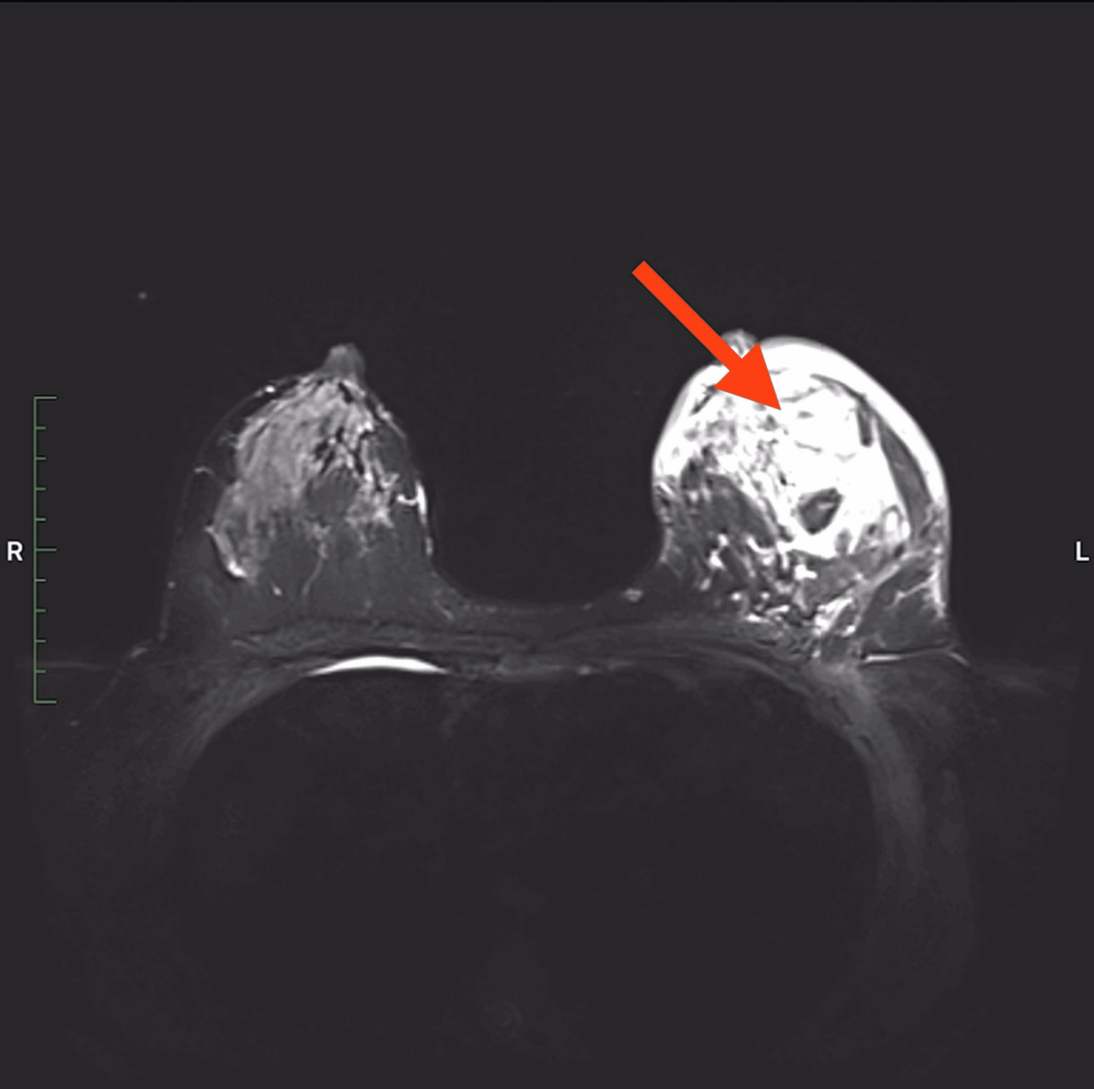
MRI of both breasts showing abscess in the left breast (red arrow) MRI: magnetic resonance imaging
